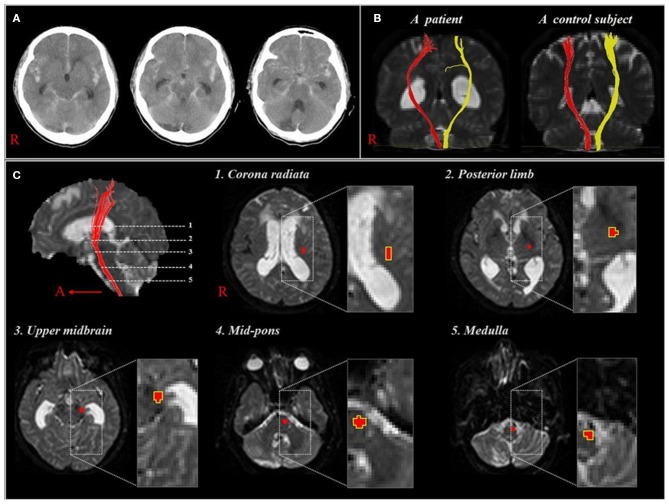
(A), CT showing a subarachnoid hemorrhage of one patient at onset (58 year-old woman). (B), Diffusion tensor tractographies for the corticospinal tract of the patient shown in (A) and one normal control subject (41 year-old man). (C), Images show 5 regions of interest along the corticospinal tract pathway [reprinted with permission from Yeo et al. (5)].
